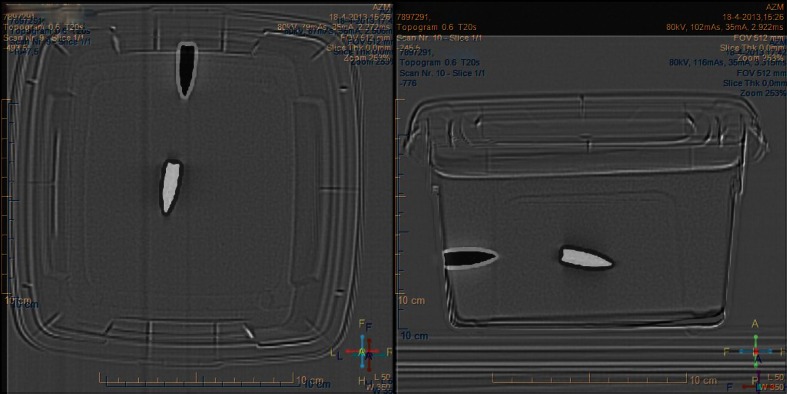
Top view (*left*) and lateral view (*right*) of a MDCT of gelatine 1. The projectile before exposure, the magnetic field of the MRI is shown in *white*; the position of the projectile after exposure to the MRI is shown in *black*. A clear displacement of the projectile has occurred along the direction of the main axis of the static MRI field
